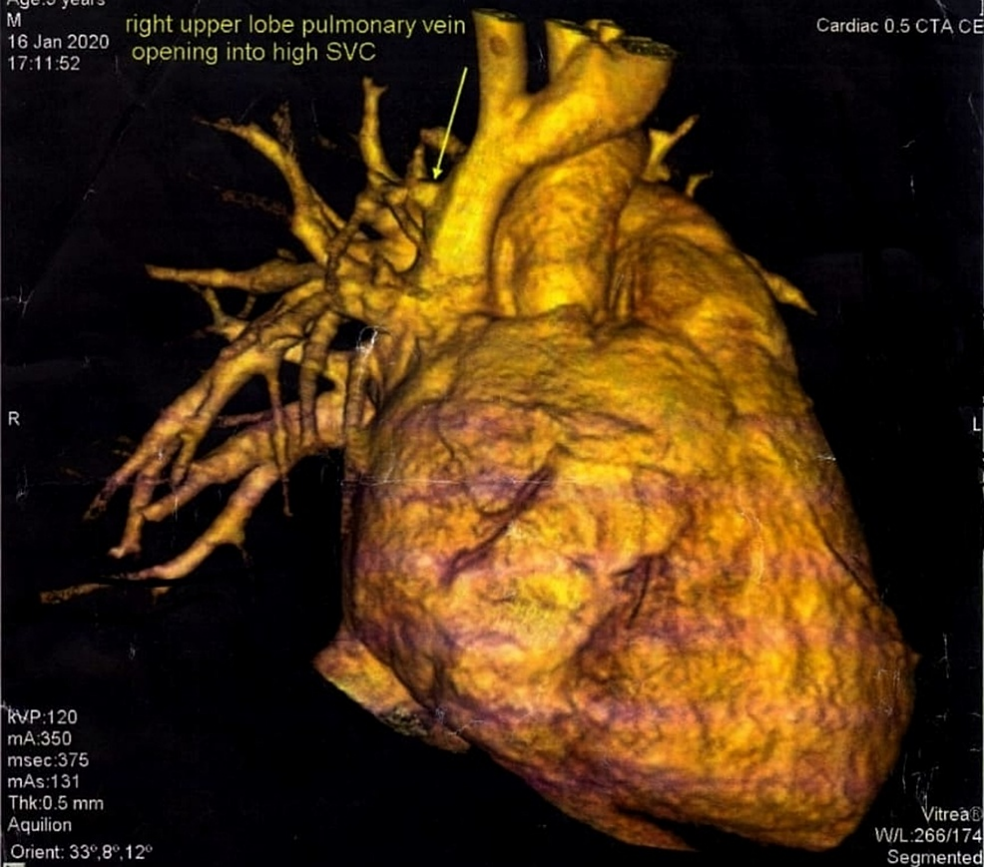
Anomalous right upper pulmonary vein opening into high right superior vena cava M: male patient, SVC: superior vena cava, CTA: computed tomography angiography, CE: contrast enhanced, R: right side, L: left side, W/L: window level.
